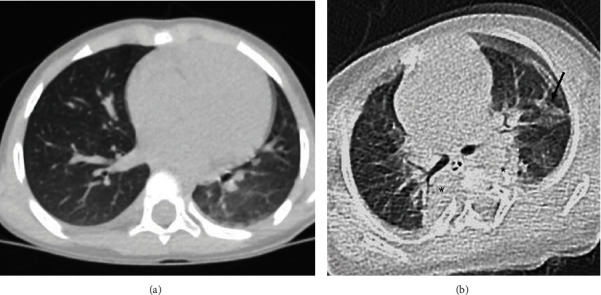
Axial CT of the chest of two different patients with positive COVID-19 RT-PCR test. (a) A 4-year-old female was presented with fever and cough. CT shows a ground-glass opacity involving the left lower lobe, which is an indeterminate chest radiographic finding of pediatric COVID-19 pneumonia. The patient clinically improved and was discharged after 10 days. (b) A 7-month-old female with biliary atresia was presented with fever and respiratory distress. CT shows bilateral patches of consolidation (asterisk) associated with a ground-glass opacity (arrow), which are typical chest radiographic findings of pediatric COVID-19 pneumonia. The patient developed progressive shortness of breath and was transferred to the intensive care unit, was mechanically ventilated, and died.
